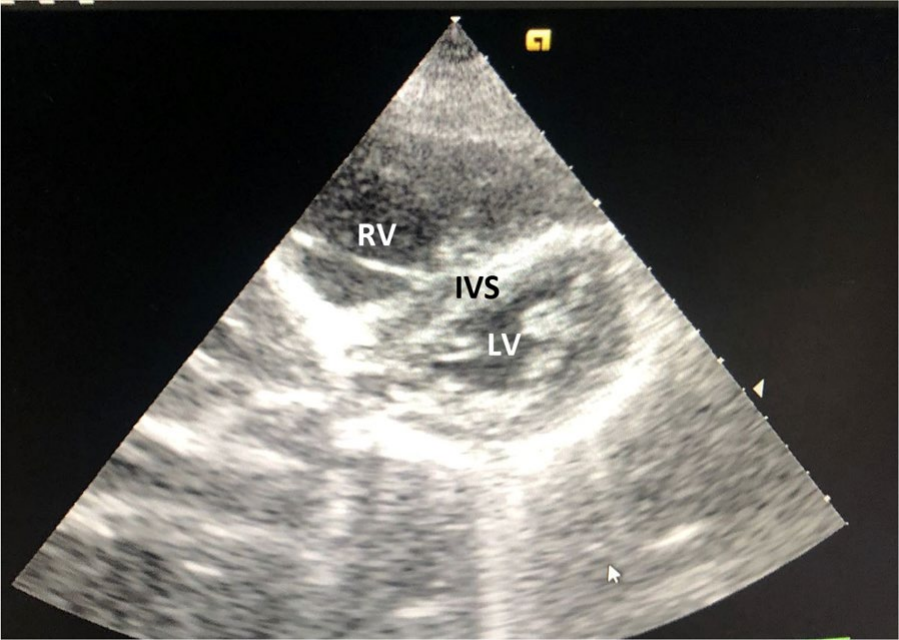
Patient echocardiogram at ER showing dilated right ventricle and D-shaped interventricular septum with systolic flattening
